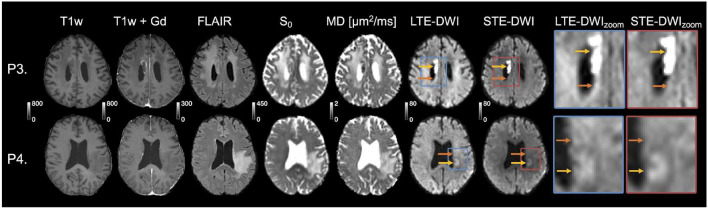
Tumor-related hyperintensities obscured by white matter. The top row (patient P3) shows images obtained to evaluate radionecrosis in a female (69 years old) diagnosed with anaplastic astrocytoma of grade III (wild-type IDH). The periventricular white matter (orange arrow) surrounding the tumor-related hyperintensity (yellow arrow) is visible with LTE-DWI, but is attenuated with STE-DWI. The hyperintensity is more clearly delineated with STE-DWI. The second row (P4) shows images obtained to confirm tumor progression in a female (71 years old) diagnosed with glioblastoma grade IV [methylated; O6-methylguanine-DNA methyltransferase (MGMT)]. A tumor-related hyperintensity (yellow arrow) can be seen with similar intensity as the surrounding white matter on LTE-DWI. With STE-DWI, the hyperintensity stands out more clearly (orange arrows) and could be missed out if only the LTE-DWI is inspected.
